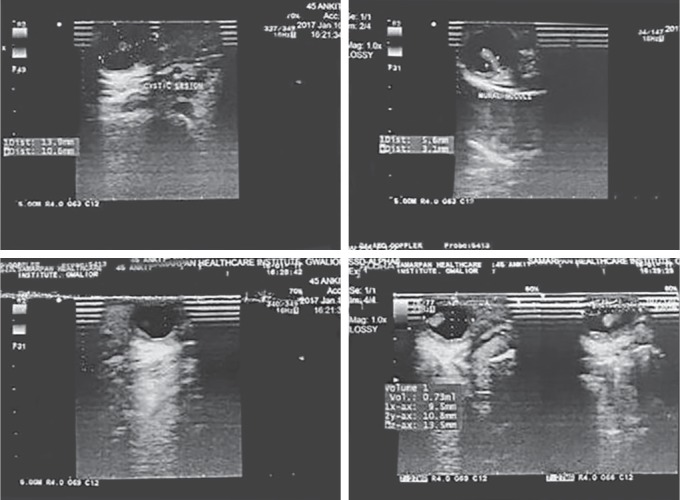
Ultrasonography of the lesion revealed a thin walled anechoic cystic lesion measuring 13 × 10 × 9 mm in the submucosal plane
